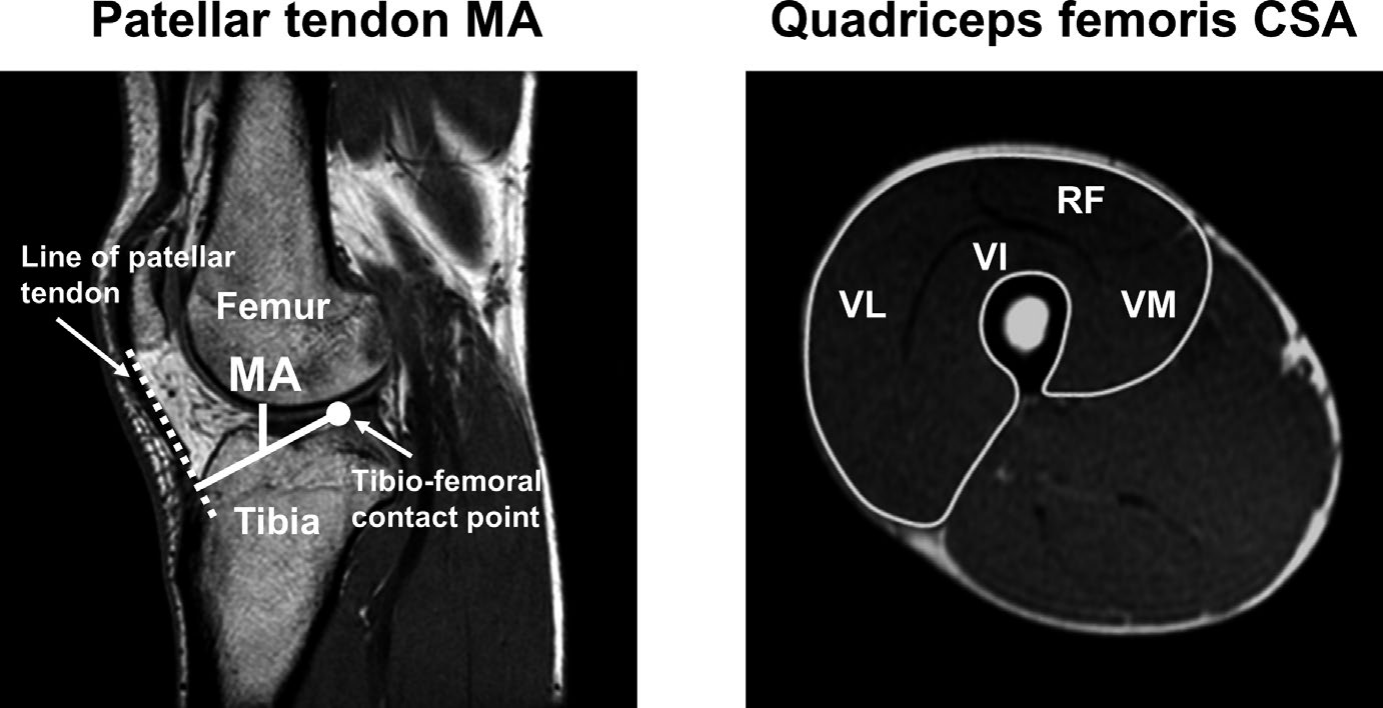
Representative magnetic resonance imaging scans for measuring the patellar tendon moment arm (MA) and quadriceps femoris muscle volume (MV). The left scan shows a sagittal image on the knee joint of the right leg. The patellar tendon MA was calculated as the distance between tibio‐femoral contact point and mid‐line of the patellar tendon. The right scan shows an axial image on the midthigh level of the right thigh. The cross‐sectional areas (CSA) of the quadriceps femoris included the rectus femoris (RF), vastus intermedius (VI), vastus lateralis (VL), and vastus medialis (VM). The quadriceps femoris MV was calculated by multiplying the sum of the CSAs along their length at intervals of 1 cm
